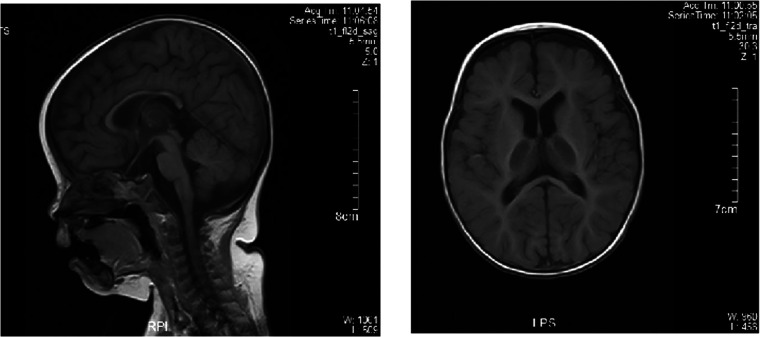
Brain MRI of the proband. Brain MRI showed the normal bilateral lateral ventricle and slightly thinner corpus callosum.
